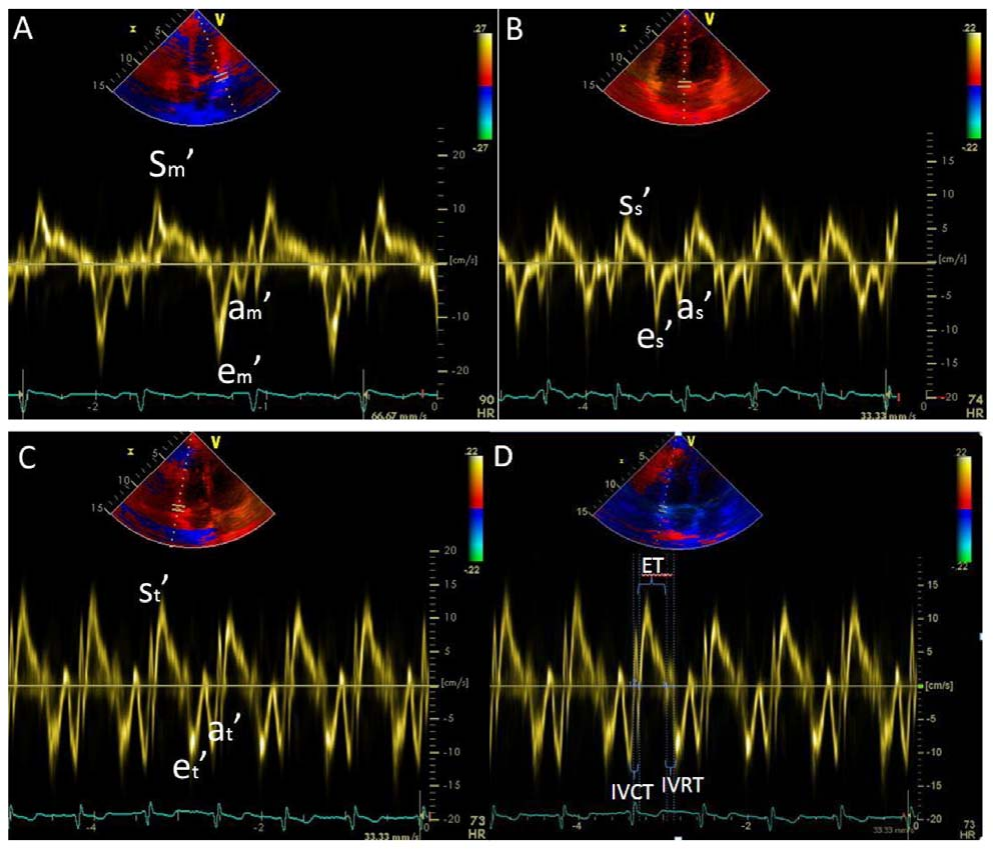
Tissue Doppler at the lateral and septal walls of the mitral annulus (A, B); Tissue Doppler at the lateral wall of the Tricuspid annulus (C); Tissue Doppler of Tei Index (D).
